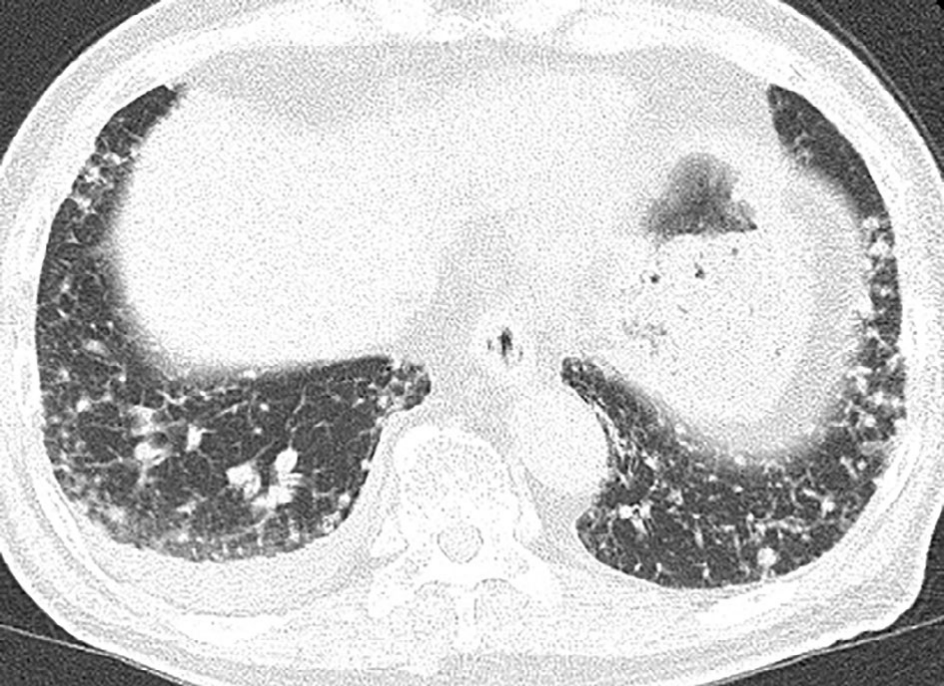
Chest computed tomography. Subpleural nodular opacities and irregular pleural thickening are seen with bilateral basal predominance. A small right‐sided pleural effusion is also observed.
